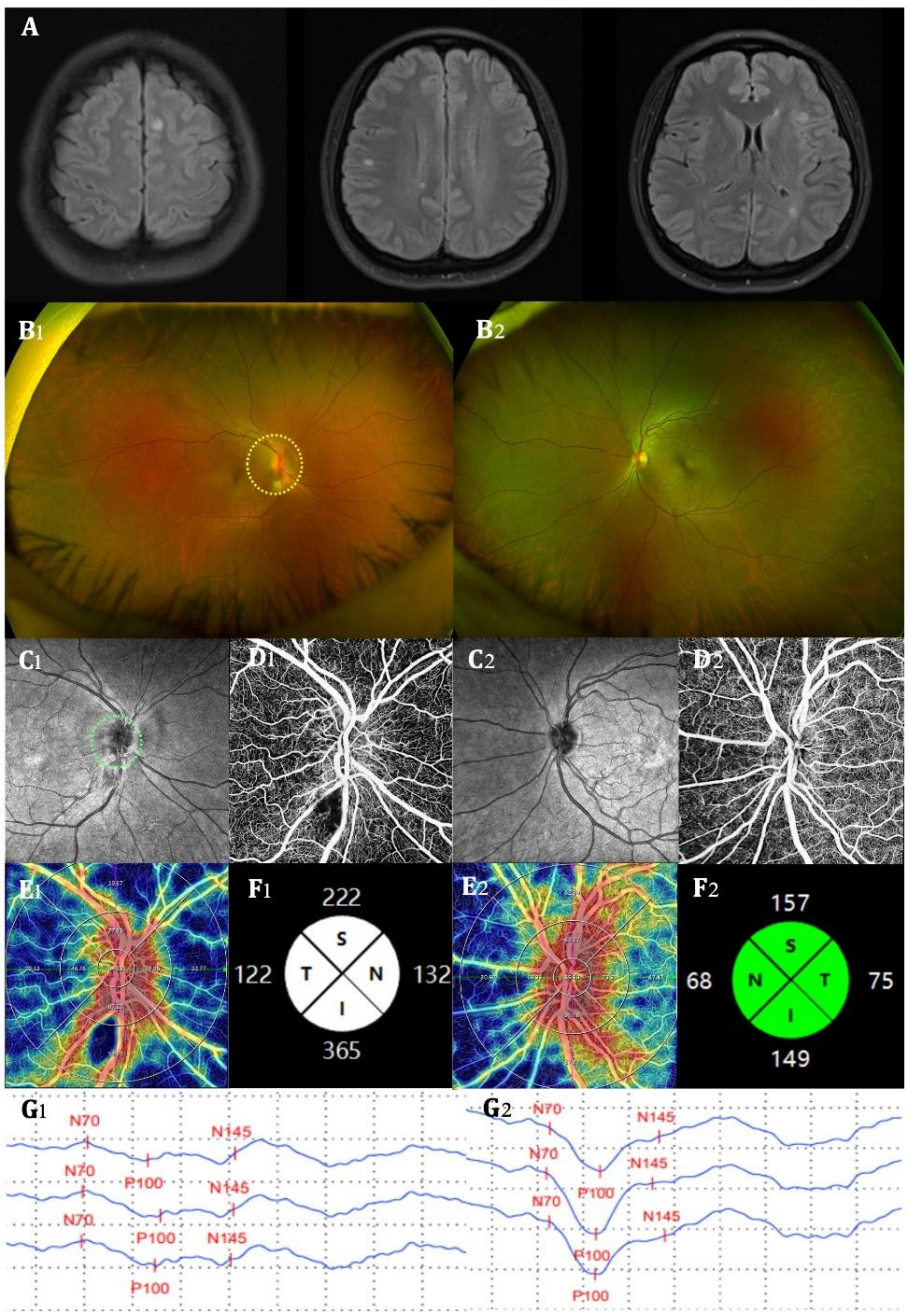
An example of OCTA findings in a MS patient. A 24-year-old female was admitted to the hospital due to “blurred right eye vision for 4 days accompanied by eye rotation pain”. The brain MRI T2-FLAIR sequence (A) showed multiple hyperintensities. Positive oligoclonal bands were found in CSF. Ultimately, the patient was diagnosed as MS. The fundus photography revealed that the right eye’s optic disc (yellow circle) was pale and edematous, with unclear boundaries compared with the left eye (B1 *vs.* B2). OCTA examination further revealed that the right eye exhibited disc edema (green circle) with indistinct boundaries (C1 *vs.* C2), unclear vascular boundaries (D1 *vs.* D2), decreased SRCP VD (E1 *vs.* E2), and increased pRNFL thickness due to edema (F1 *vs.* F2) compared with the left eye. The VEP test showed a decrease in the amplitude of the right eye compared to the left eye (G1 *vs.* G2). MRI, magnetic resonance imaging; CSF, cerebrospinal fluid; MS multiple sclerosis; OCTA, optical coherence tomography angiography; pRNFL, peripapillary retinal nerve fiber layer; SRCP, superficial retinal capillary plexus; VD, vessel density; VEP, visual evoked potential. N, nasal; T, temporal; S, superior; I, inferior.
